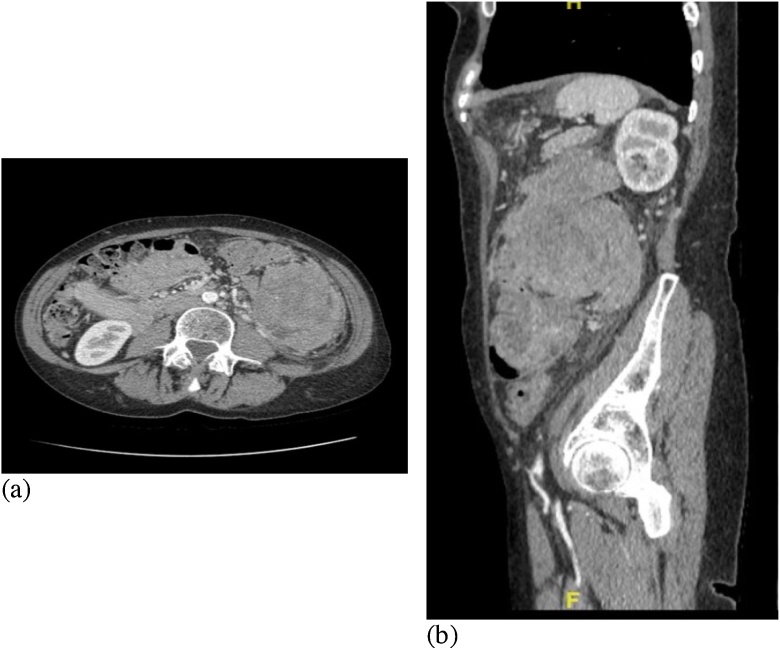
CT Scans with IV contrast; (a) shows the axial view revealing a large polylobulated mass in the left abdomen, and (b) shows the sagittal view revealing a large mass occupying most of the left abdomen.
